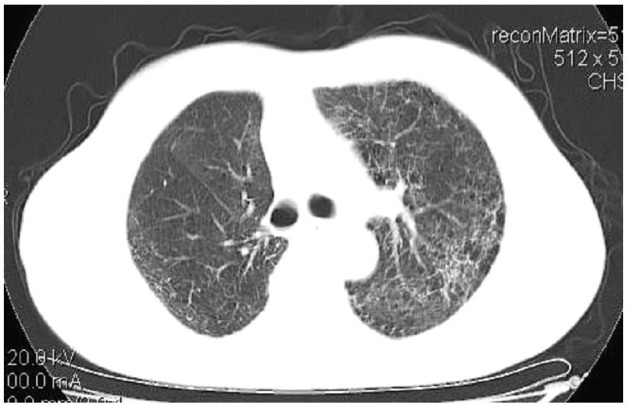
Chest computed tomography (CT) scan.
